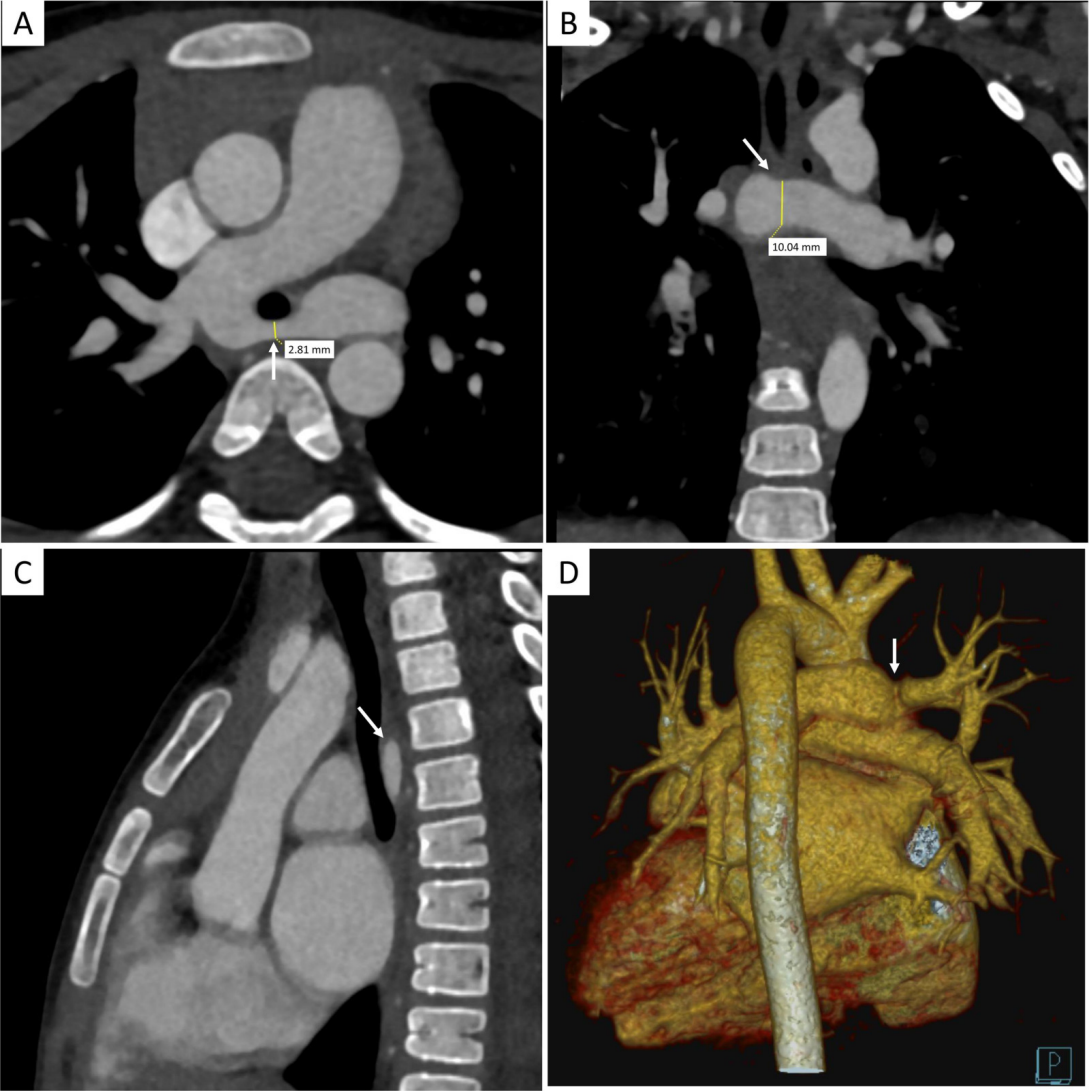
Preoperative multiplanar contrast-enhanced computed tomography angiography (CTA) showing LPA stenosis. (A) Axial view demonstrating the severe focal stenosis at the LPA origin, with a minimal anteroposterior diameter of 2.8 mm (yellow line). (B) Coronal reformatted view showing the wider craniocaudal dimension of 10.0 mm at the same level (yellow line), revealing the slit-like nature of the stenosis. (C) Sagittal view showing the LPA (arrow) coursing between the trachea and esophagus. (D) 3D VR reconstruction highlighting the LPA origin (arrow) and its relationship with the great vessels.
